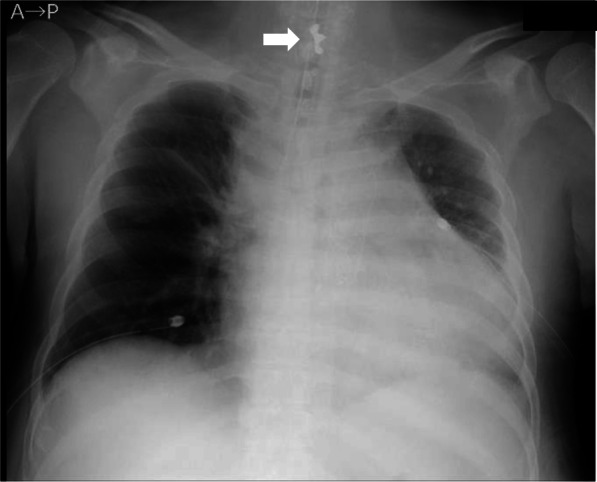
A chest radiograph taken on POD 34. A foreign body was confirmed at the midline of the neck (arrow). POD, postoperative day
